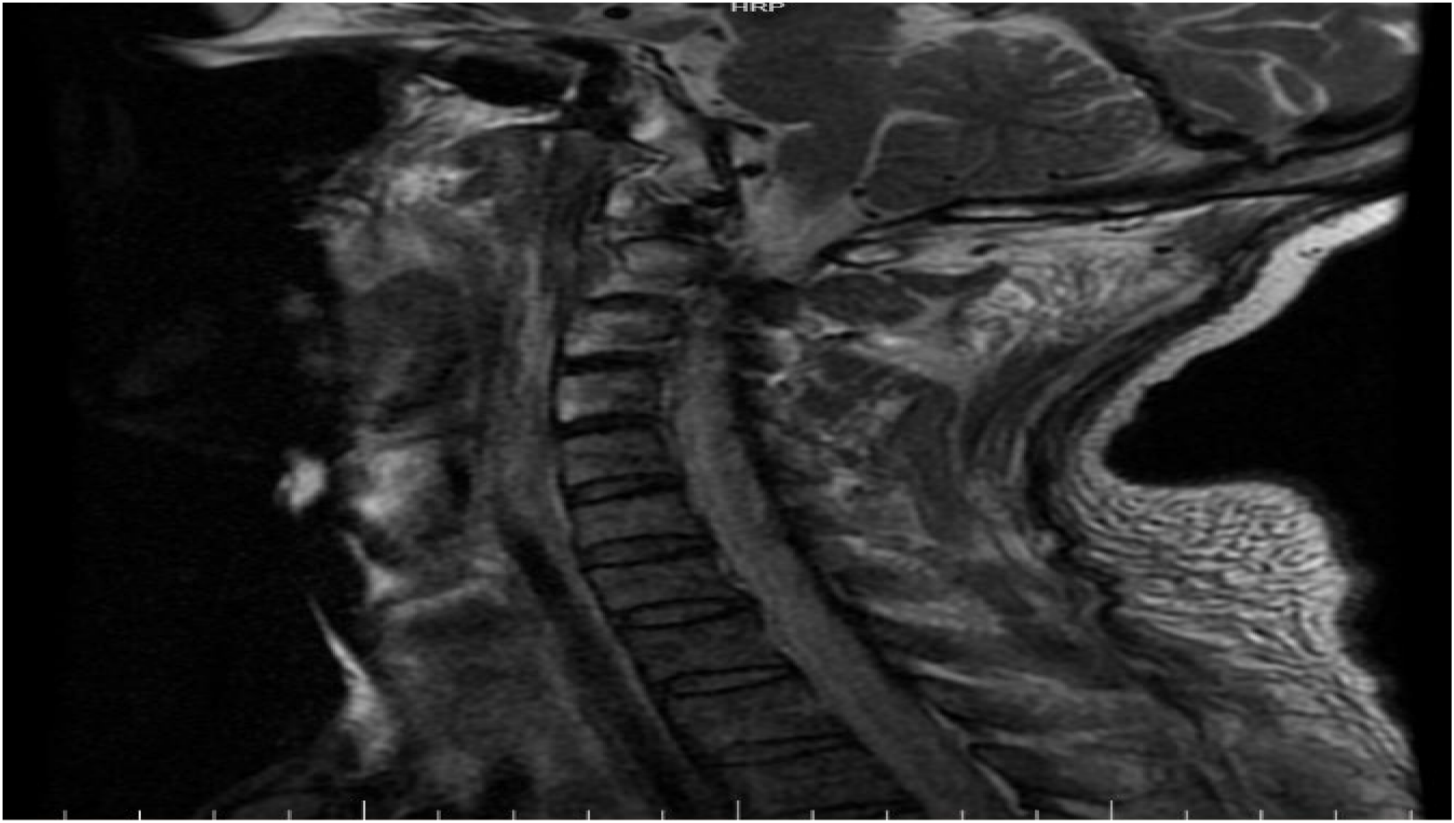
The cervical MRI findings further support active dermatomyositis, with combined posterior cervical muscle and anterior fascial involvement representing a typical imaging pattern of systemic proximal myositis with fascial inflammation. These findings are consistent with the lower-extremity MRI, indicating widespread active disease.
